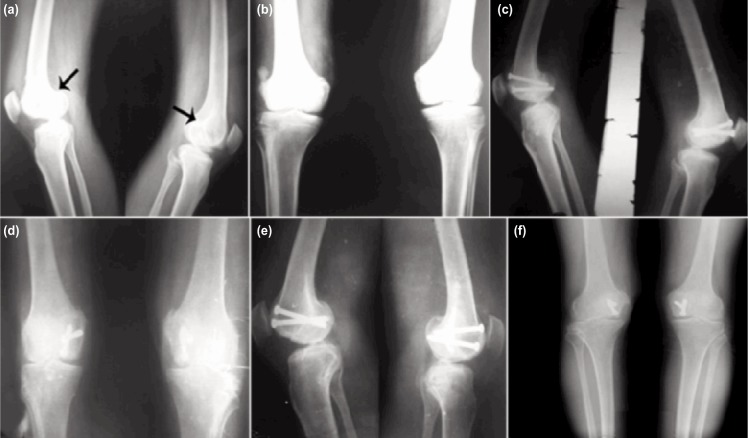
(a, b) Case with bilateral Hoffa fracture involving medial condyle on both side with (black arrow), (c, d) Plain radiographs performed at 8 weeks showing fracture union and (e, f) Radiological union was achieved by the end of 16 week.
